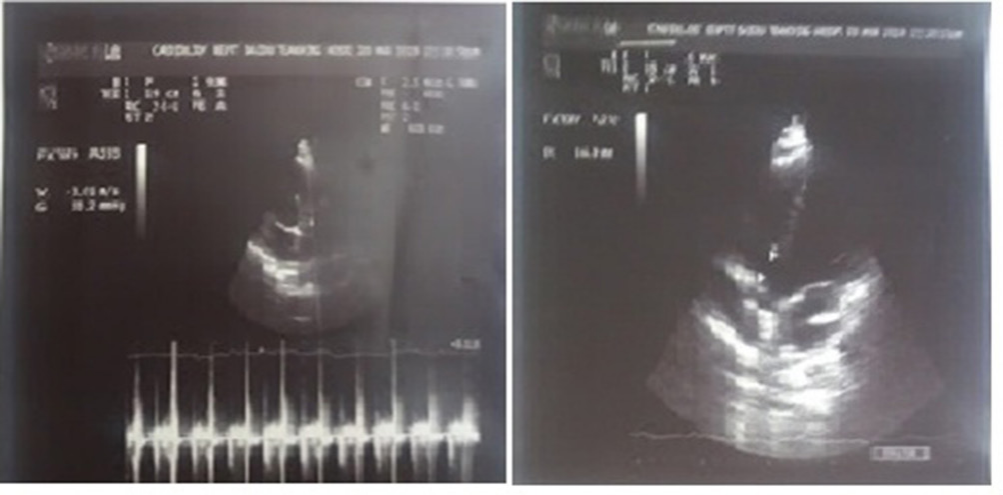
Transthoracic echocardiography showing a vegetation in the pulmonary valve and ventralseptal defect.
